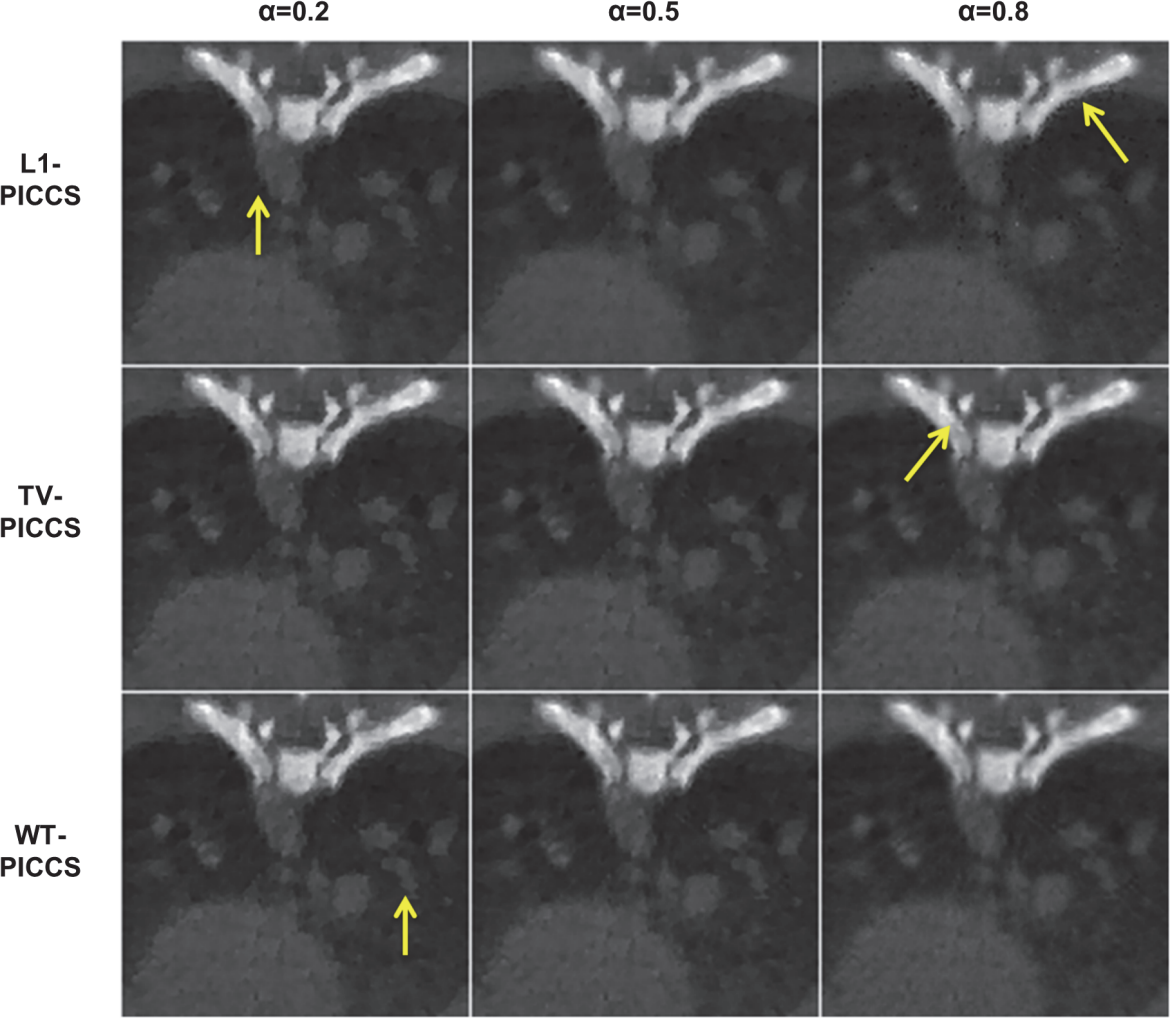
Zoomed-in images of the low-dose protocol data for respiratory phase one. Reconstructions were performed with L1-PICCS, TV-PICCS, and WT-PICCS for α equal to 0.2, 0.5, and 0.8, for 120 projections and X-ray flux corresponding to a number of photons *I*
